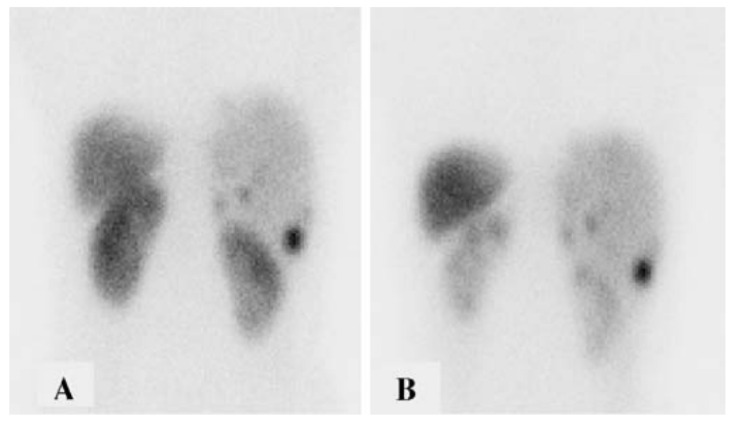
Abdominal scintigraphy in one patient after 220 MBq of [^111^In]In-DTPA^0^-octreotide. The images were obtained without (A) and with (B) co-infusion of 75 g of lysine. Renal radioactivity was 59% of control when lysine was infused. Reprinted with permission from ref. [32]. Copyright 2003 Springer-Verlag.
